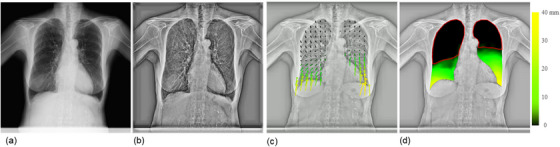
An example of a false‐positive case. Frame of the original dynamic chest radiography (DCR) images (a), bone‐suppressed DCR with enhanced high spatial frequency (b), vector‐map images (c), and displacement‐map image (d) of a 78‐year‐old female with right lung cancer (grade 0). The patient showed poor motion in 64.3% and 49.1% of the area of the right and left lungs, respectively; the predicted VC was 107.2%, predicted FEV1 was 124.0%, FEV1/FVC ratio was 69.2, and predicted DLco was 54.4%. DLco, diffusing capacity of the lung for carbon monoxide; FEV1, forced expiratory volume in 1 s; FEV1/FVC, forced expiratory volume in 1 s/forced vital capacity; VC, vital capacity.
